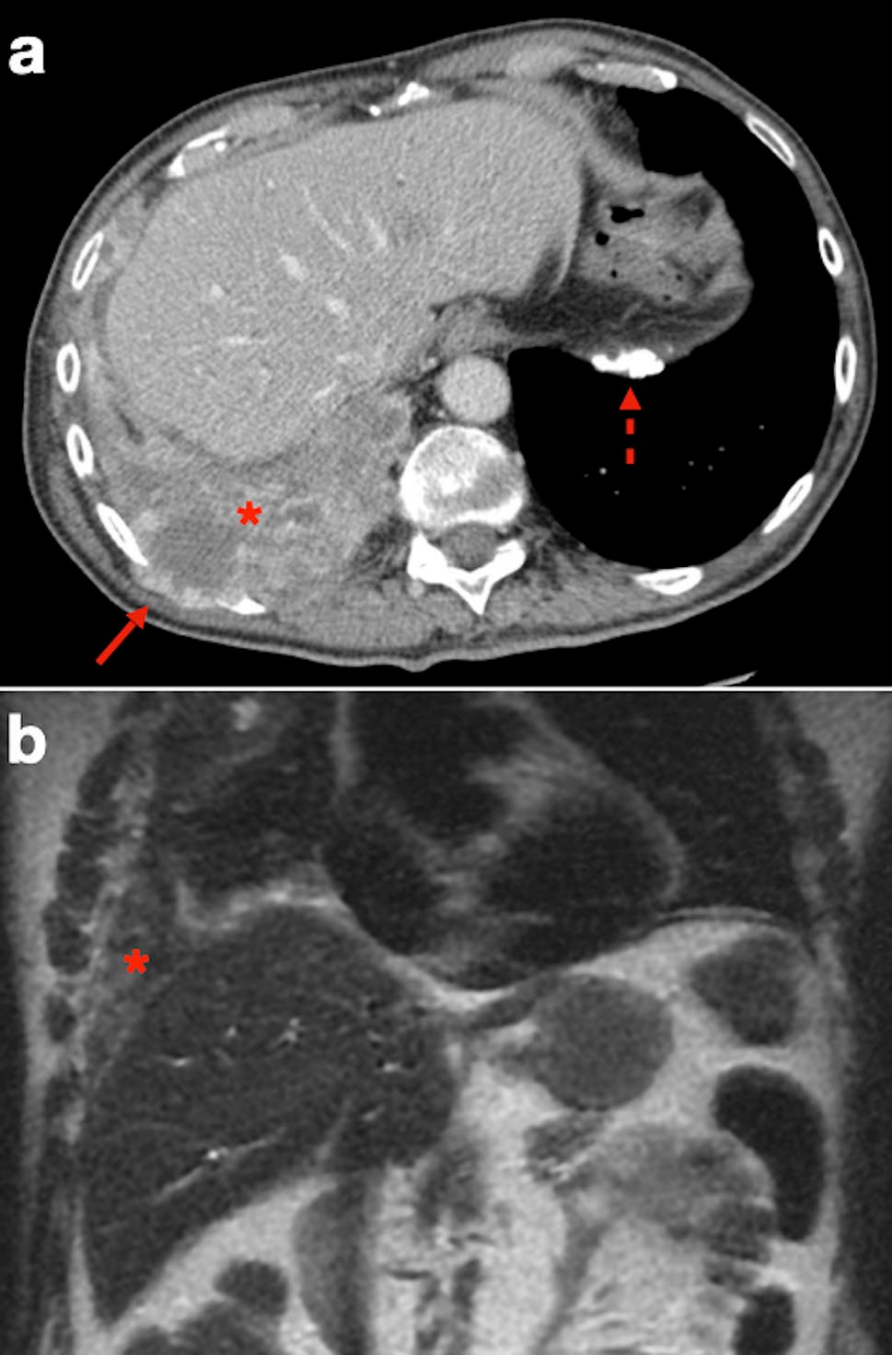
69-year-old man with history of asbestos exposure and locally advanced PM. a CT image shows large soft tissue mass (asterisk) that cannot be separated from the right hemidiaphragm. Infiltration of the posterior chest wall is also present (arrow). Note left-sided calcified diaphragmatic pleural plaque (dashed arrow) related to long-standing asbestos exposure. b Coronal T2-weighted MR image shows inhomogeneously hyperintense tissue based on the right diaphragmatic pleura with cancellation of the nearby subdiaphragmatic fat plane (asterisk)
